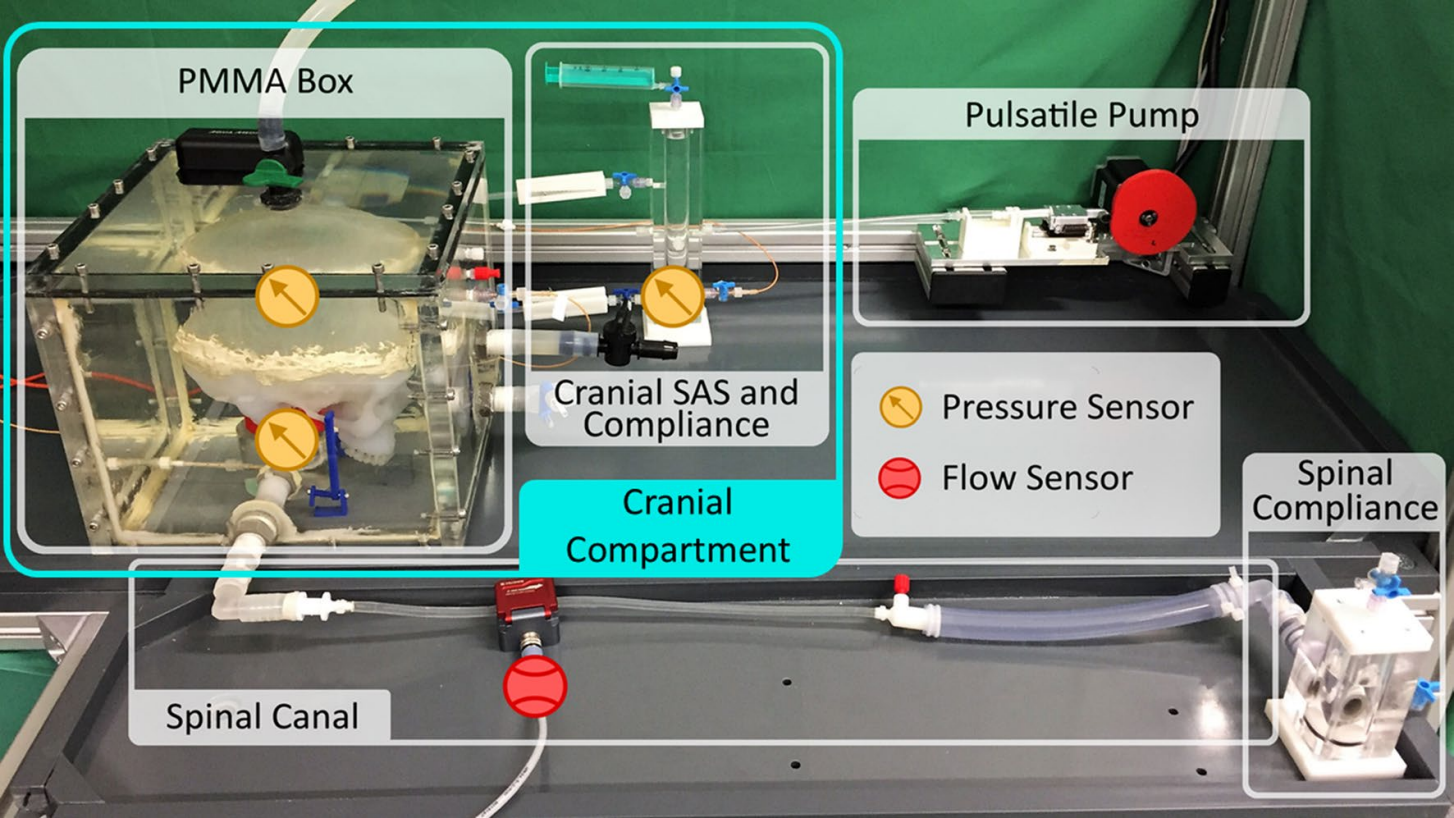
Experimental lab test bench. Three tip catheter pressure sensors (yellow) are placed inside the silicone parenchyma (measurement of ICP), the cistern and the compliance chamber of the cranial SAS. The ultrasound flow sensor (red) is attached at the upper part of the spinal canal (corresponding to the C2–C3 level)
